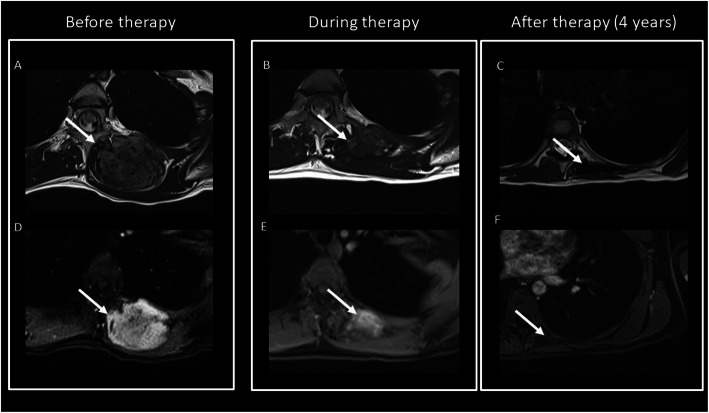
Chest posterior wall DT (T2wi (a) and after contrast T1wi (d)). A 23-year-old female patient underwent chemotherapy. MRI showed progressive decrease of T2wi signal intensity and contrast enhancement on T1wi of the lesion (white arrow): during therapy, the lesion decreased in dimension and in T2 hyperintensity (b) but maintained moderate contrast enhancement on T1wi (e). After 4 years, the lesion significantly reduced its dimension, become hypointense on T2wi (c), and did not show significant contrast enhancement (f)
